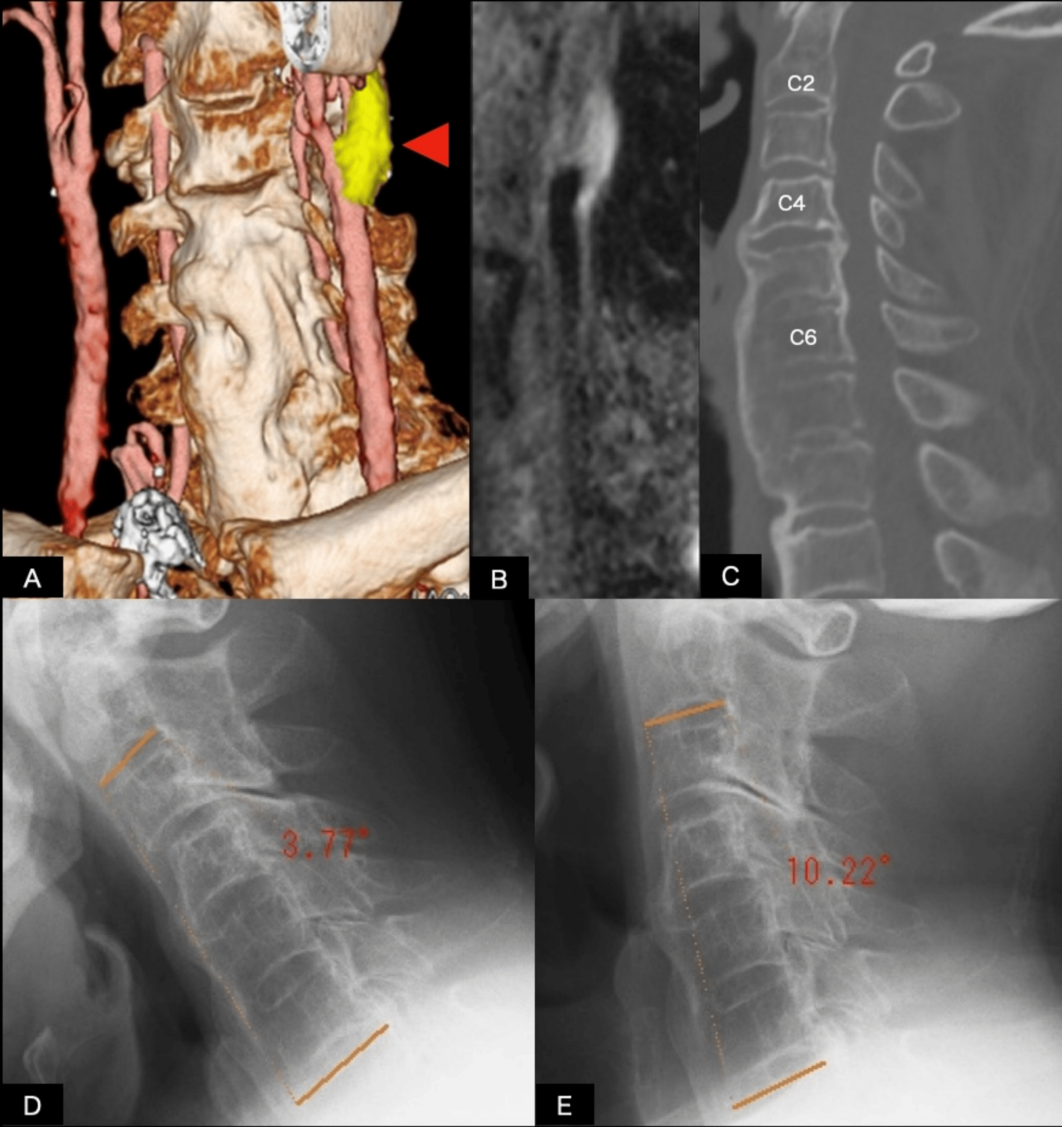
Preoperative multimodal imaging and evaluation of cervical spine immobility in Case 2 (A) Fused non-contrast CT and MRA image of Case 2 shows the common carotid artery bifurcation and plaque (arrowhead). Anterior bony bridging of the spine is also demonstrated; (B) black-blood fat-suppressed T1-weighted MRI demonstrates hyperintensity consistent with intraplaque hemorrhage and increased plaque vulnerability; (C) sagittal CT of the neck shows DISH below the C4 level and OPLL from C2 to C6 levels; (D, E) lateral cervical radiographs obtained in flexion (D) and extension (E). The angle between the inferior endplate of C2 and the superior endplate of C7 measured −3.77° in flexion and 10.22° in extension, crossing 0° between positions. The estimated flexion-extension range of motion was 13.99° (3.77° + 10.22°), demonstrating limited dynamic change. CT: computed tomography; MRA: magnetic resonance angiography; DISH: diffuse idiopathic skeletal hyperostosis; OPLL: ossification of the posterior longitudinal ligament
